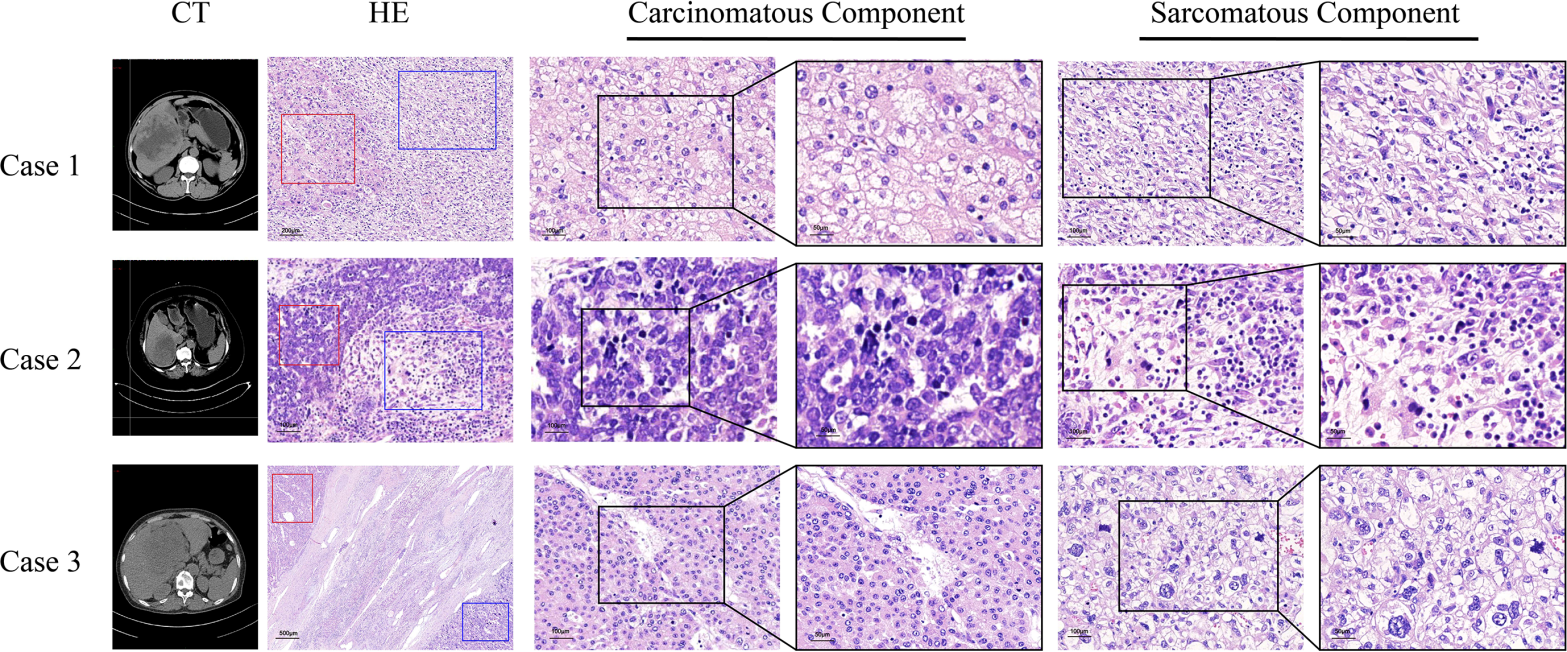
Computed tomography scans, the morphology of sarcomatous components and carcinomatous components in the tissues of three HCS cases. Red frames represent carcinomatous components and blue frames represent sarcomatous components.
